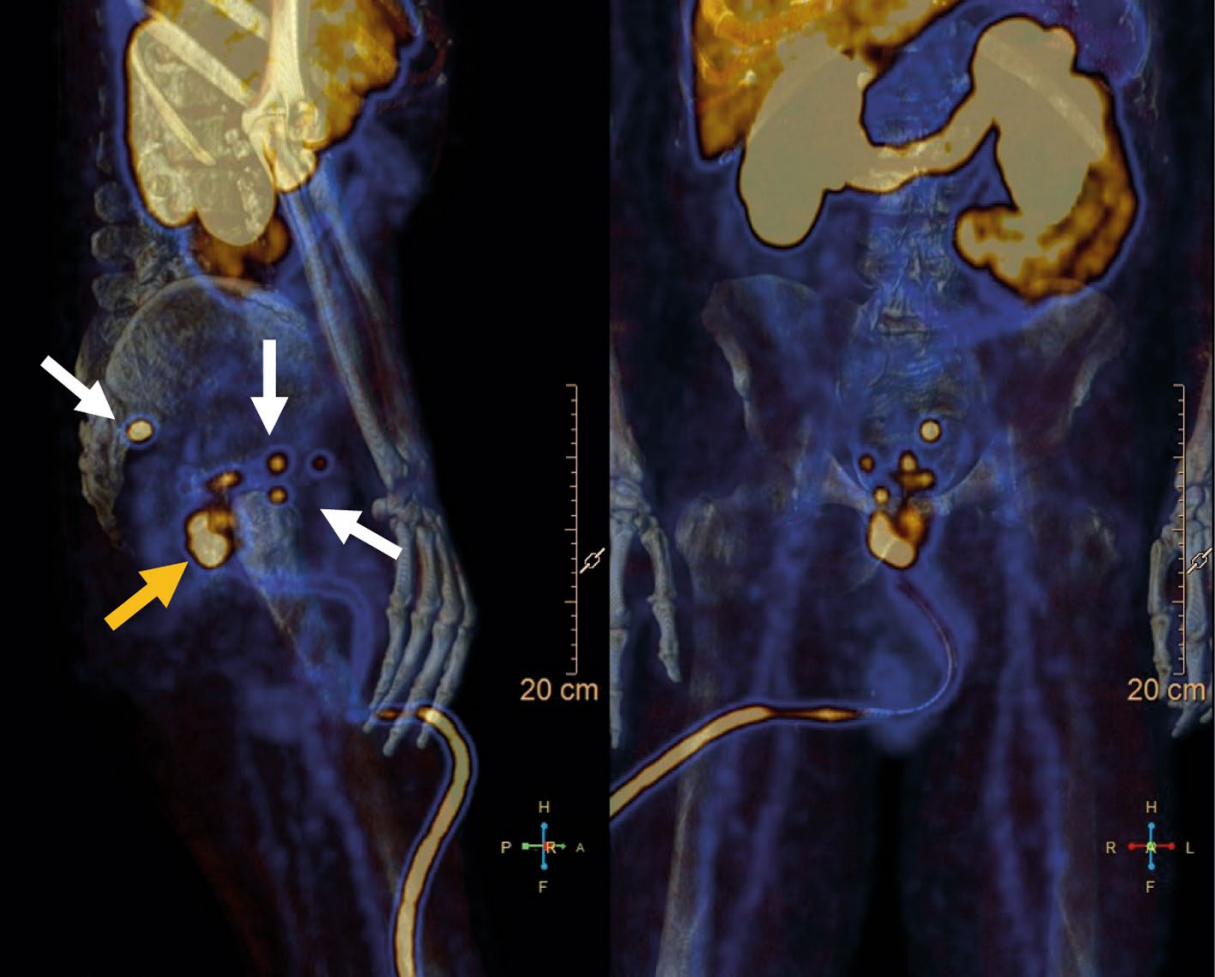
Patient 01 pre-surgery PSMA PET/CT: the white arrows represent the pelvic lymph nodes; the yellow arrow represents the prostate lesion
